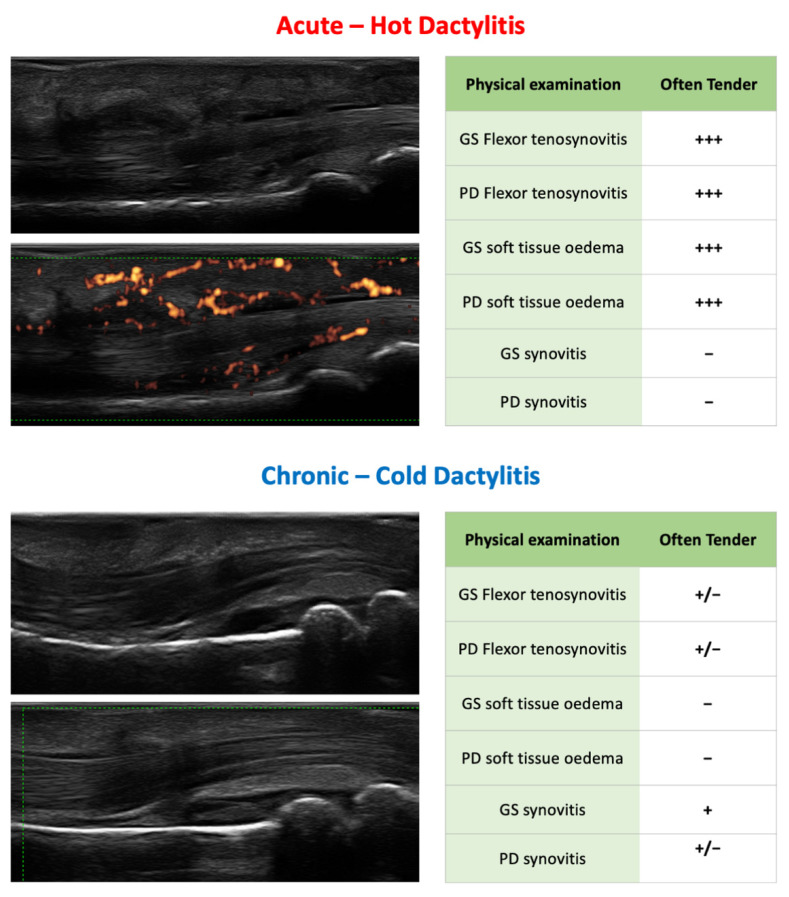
This figure summarizes the clinical and ultrasound characteristics of acute/hot and chronic/cold forms of dactylitis: Flexor tenosynovitis and soft tissue oedema are predominant in early cases, whereas synovitis is more frequent in the chronic form. Similarly, local symptoms are linked with extracapsular lesions, particularly flexor tenosynovitis and soft tissue oedema; joint synovitis does not seem to correlate with pain.
